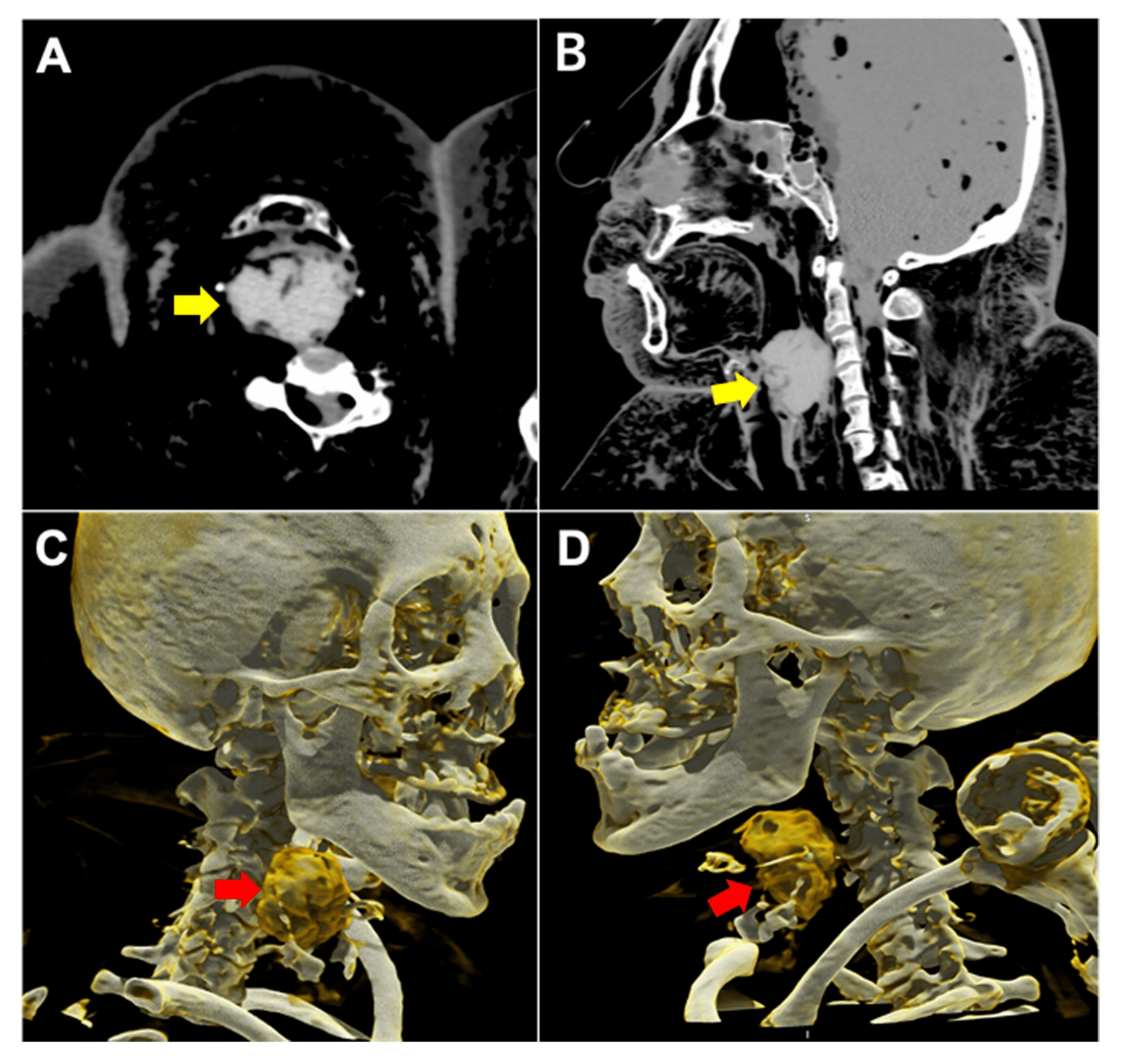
PMCT findings (A, B) Two-dimensional CT images of the head and neck region. (A) Axial view. (B) Sagittal view. A high-density object is observed in the pharynx, indicated by the yellow arrows. Cinematic rendering images of the (C) right lateral view and (D) left lateral view. The foreign body in the pharynx appears yellow-brown, indicated by red arrows. The images were reconstructed using OsiriX MD software. PMCT: Postmortem CT
